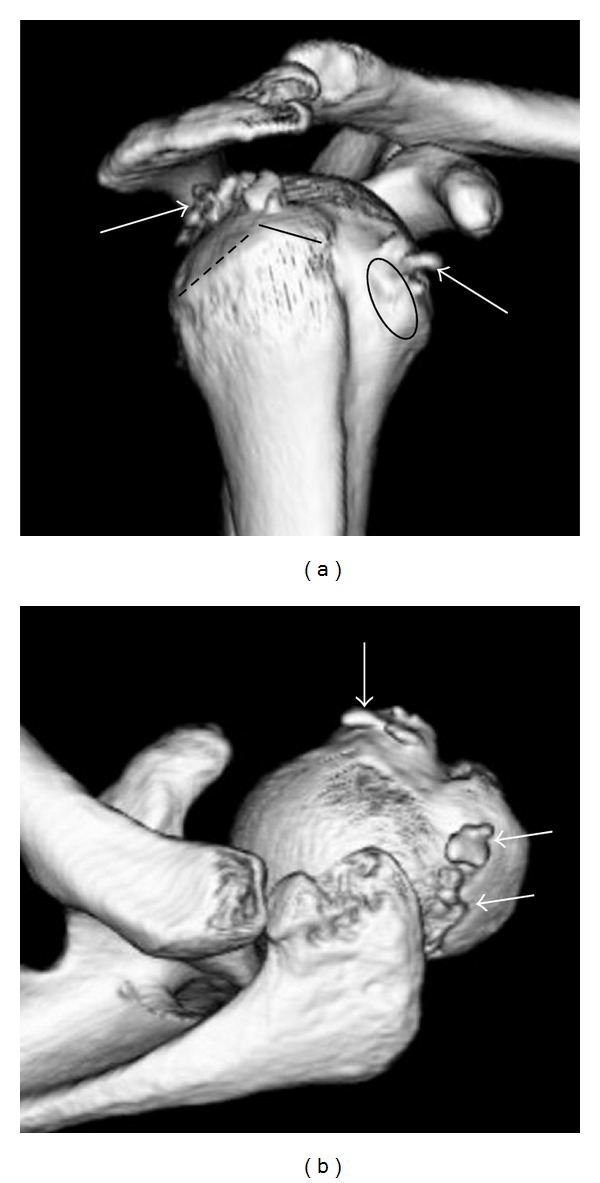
(a) and (b) Three-dimensional computed tomographs showing calcific deposits at the lesser tuberosity and the middle facet of the greater tuberosity. White arrows indicate the presence of calcific deposit. Solid line: superior facet, broken line: middle facet, circle: lesser tuberosity.
